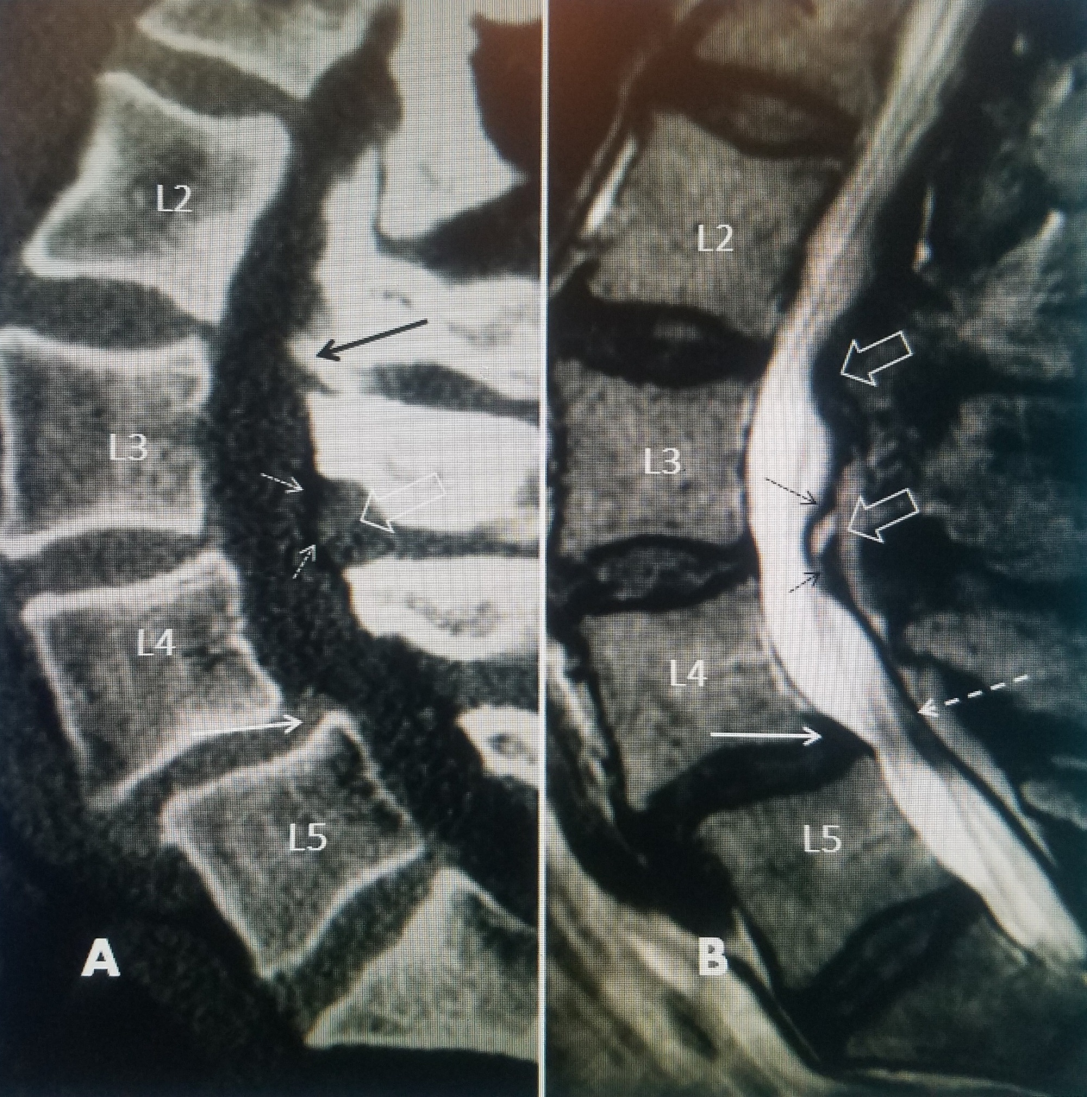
Comparison of computerized tomography (CT) scan and magnetic resonance imaging (MRI) scan in same patient. A: Sagittal CT scan showing L4-5 spondylolisthesis (solid white arrow). At L3-4 there is a mild posterior compression (open white arrow) from ligament hypertrophy into the posterior epidural space (dashed white arrow). There is mild bone compression at L2-3 (solid black arrow). B: Sagittal MRI in the same patient clearly shows there is posterior compression at L4-5 (dashed white arrow) separate from the anteriolisthesis at L4-5 (solid white arrow). The combination of both anterior spondylolisthesis and posterior compression at the same level is causing marked canal narrowing and stenosis at L4-5. The MRI also shows that there is more obvious posterior soft tissue compression at L3-4 (dashed black arrows) as well as additional posterior compression at L2-3 and L3-4 from ligamentous and facet hypertrophy (open white arrows).
